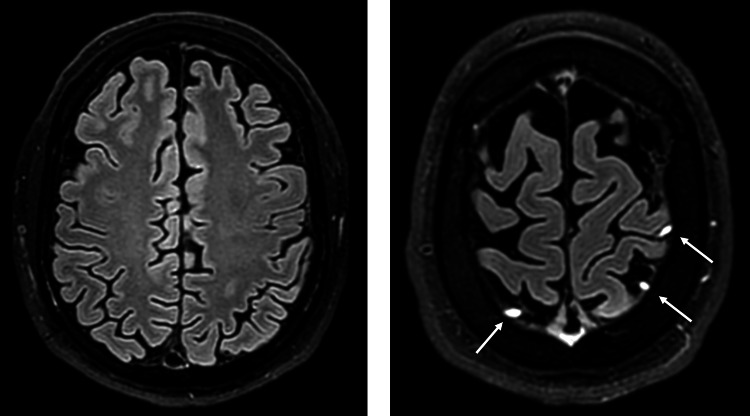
The third brain MRI (01/11/24) image showed the resolution of T2/fluid-attenuated inversion recovery (FLAIR) hyperintensity in the image on the left. The right-sided image also showed T2/FLAIR hyperintensity in regions previously corresponding to areas of thrombus.
